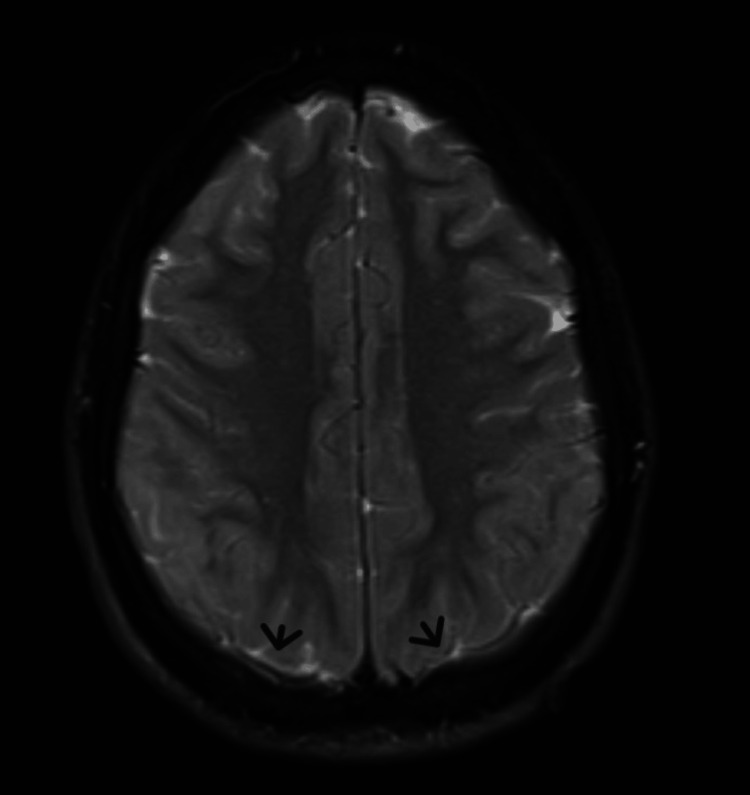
Axial MRI of the brain The scan image is demonstrating mild leptomeningeal enhancement (black arrows).
